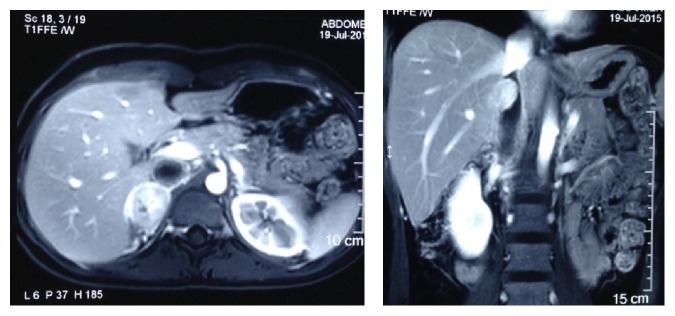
Gadolinium enhanced MRI abdomen axial and coronal view showing right adrenal mass and IVC thrombosis.
